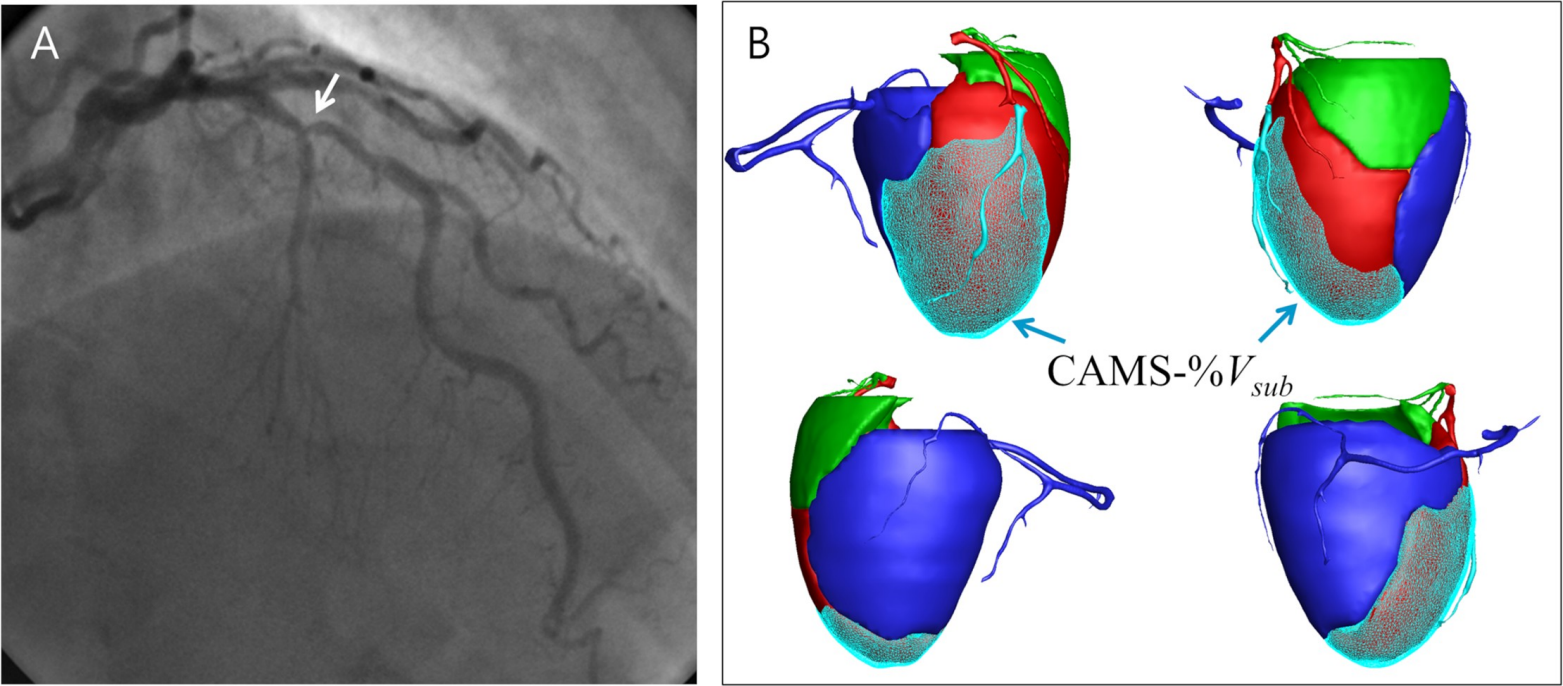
(A) Angiography shows an intermediate stenosis (white arrow) of the mid LAD. (B) The CAMS-derived myocardial volume supplied by LAD was 38.5 cc (shown as red area), and the total left ventricular myocardial volume was 110 cc. The CAMS-%LAD was 35.0%. The myocardial volume subtended to the poststenotic segment was 29.0 cc (blue arrows), and the CAMS-%*V*_*sub*_ was 26.3%. The FFR was 0.82. CAMS, coronary computed tomography angiography–based myocardial segmentation; CAMS-%*V*_*sub*_, CAMS-derived percent myocardial volume subtended to a stenotic segment; FFR, fractional flow reserve; LAD, left anterior descending.
